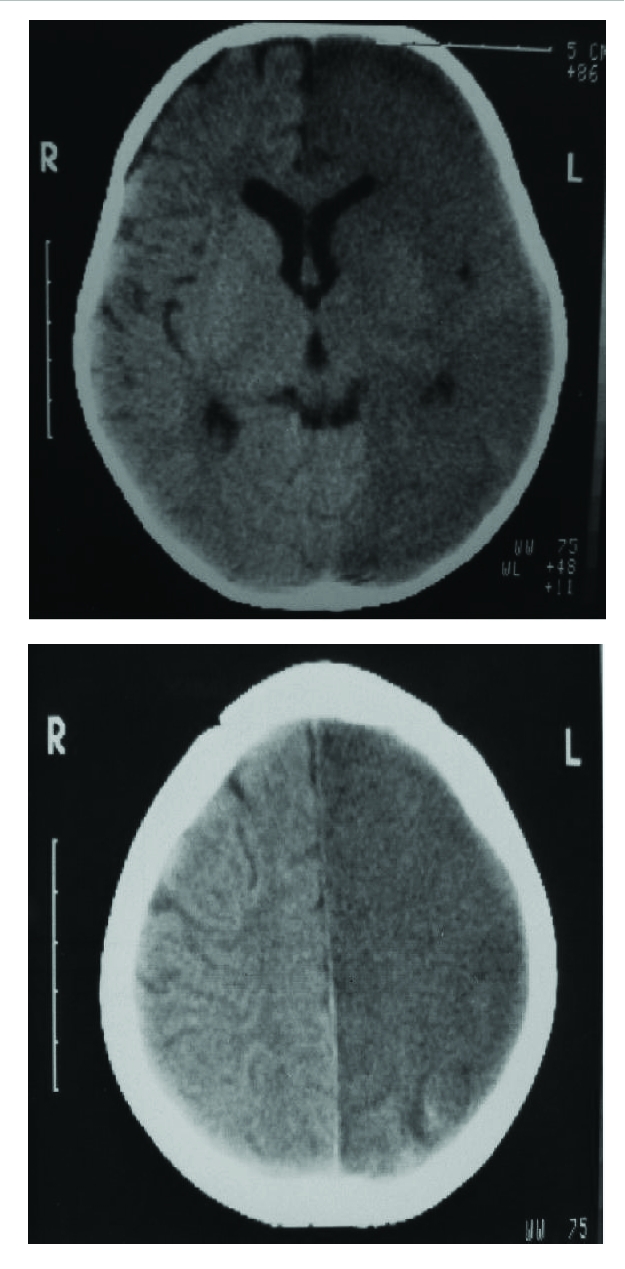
Initial Cranial CT shows total left– sided encephalomalasia
